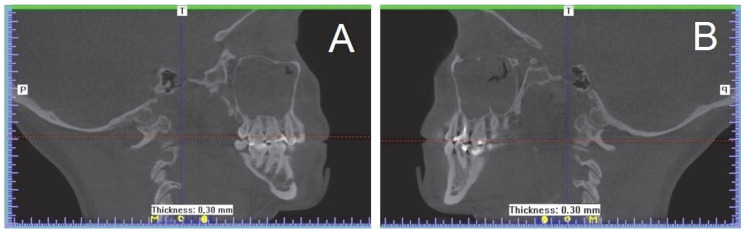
Maxillary sinuses in sagittal view: (A) Right and (B) left. (CBCT scans).
